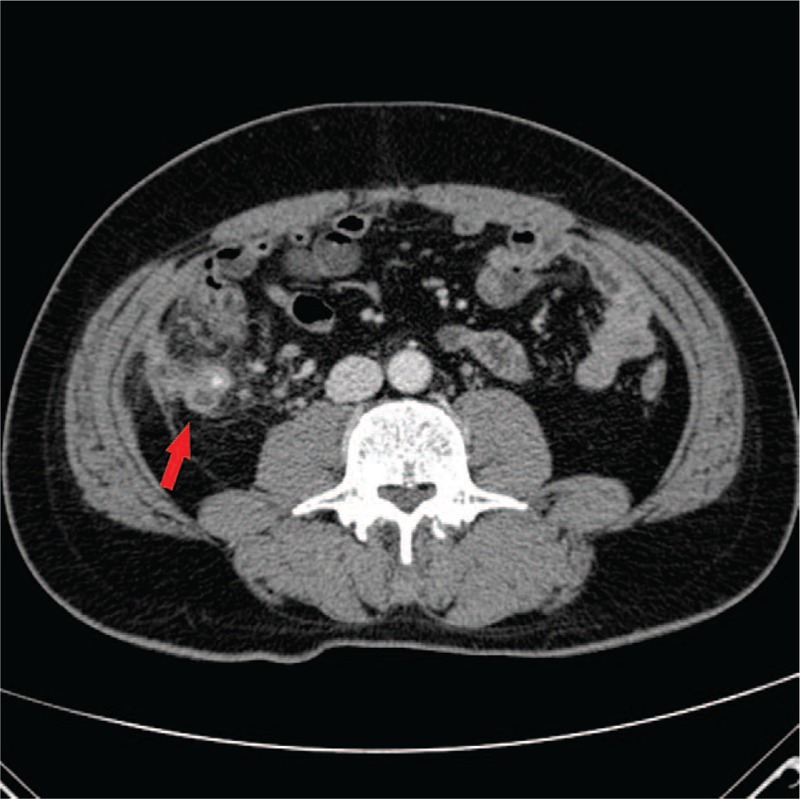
Contrast-enhanced abdominal and pelvic computed tomography in case 1. An appendicolith in the short stump appendix with inflammation around the appendix (arrow).
